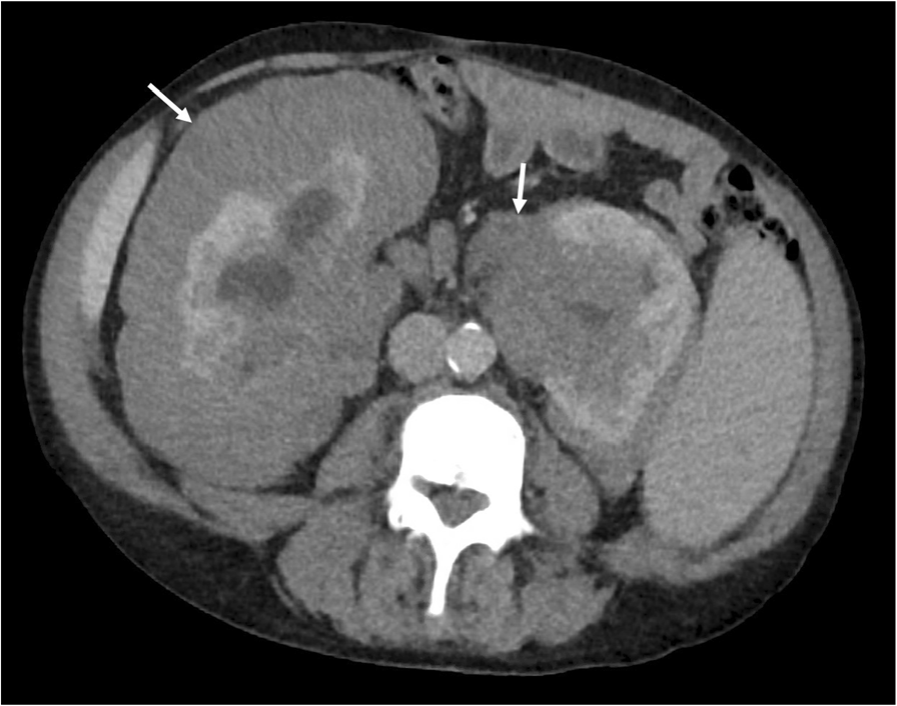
Axial CT demonstrating bilateral lobular perirenal soft tissue masses, contained by Gerota’s fascia and often not causing contour deformity against the kidneys (white arrows), consistent with perirenal extramedullary haematopoiesis, a common site of extramedullary haematopoiesis in the abdomen
